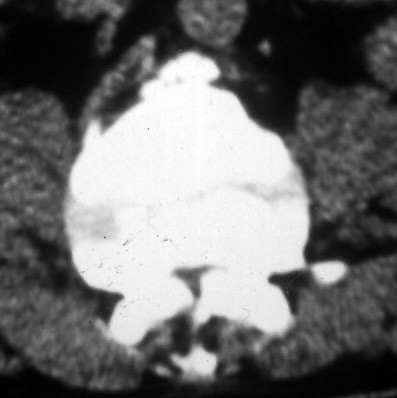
CT-scan of the spine showing severe spinal stenosis.
